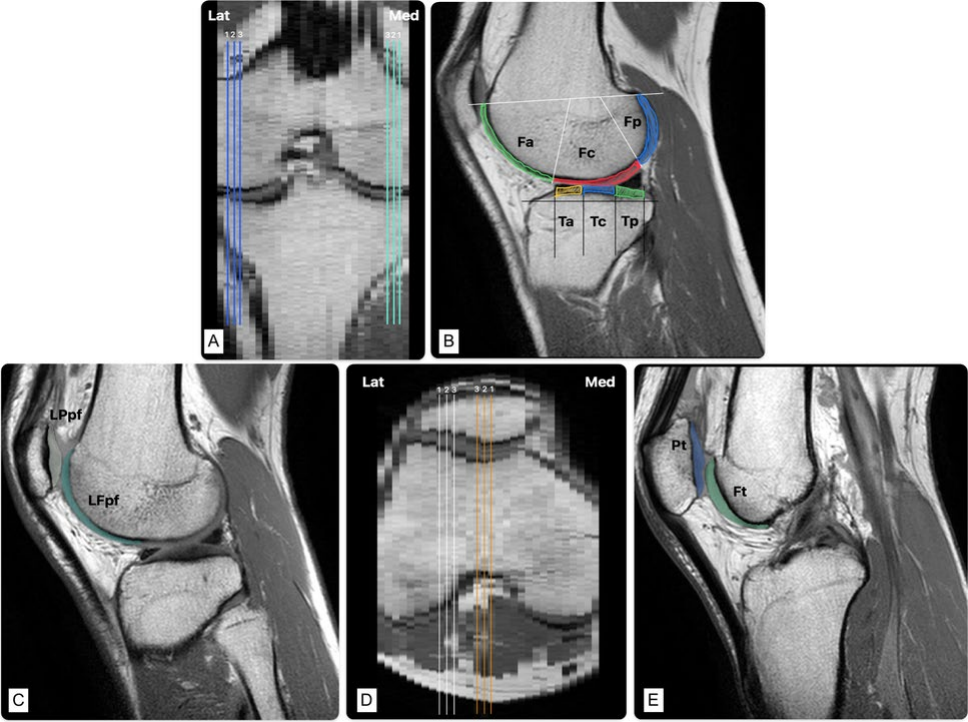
Slice ordering and KAC section definition. A and D Example of slice ordering on 3 contiguous central slices selected for segmentation of the knee cartilage, from the outermost to the innermost slice. B Whole-Organ Magnetic Resonance Imaging Score. Using the WORMS classification as a reference, the cartilage was subdivided in each sagittal image by its anatomical landmarks. The femoral articular surface was divided into medial condyle (MF) and lateral condyle (LF). Each condyle was further subdivided into three regions: anterior (a), central (c), and posterior (p). The medial tibial plateau (MT) and lateral tibial plateau (LT) are also divided into three regions: anterior (a), central (c), and posterior (p). C and E Example of the patellofemoral subdivisions. *Lat* lateral, *Med* medial, *Fa* femoral anterior, *Fc* femoral central, *Fp* femoral posterior, *Ta* tibial anterior, *Tc* tibial central, *Tp* tibial posterior, *LPpf* lateral patella of patellofemoral, *LFpf* lateral femur of patellofemoral, *Pt* patella of the trochlea, *Ft* femur of the trochlea. Source: investigator’s dataset
